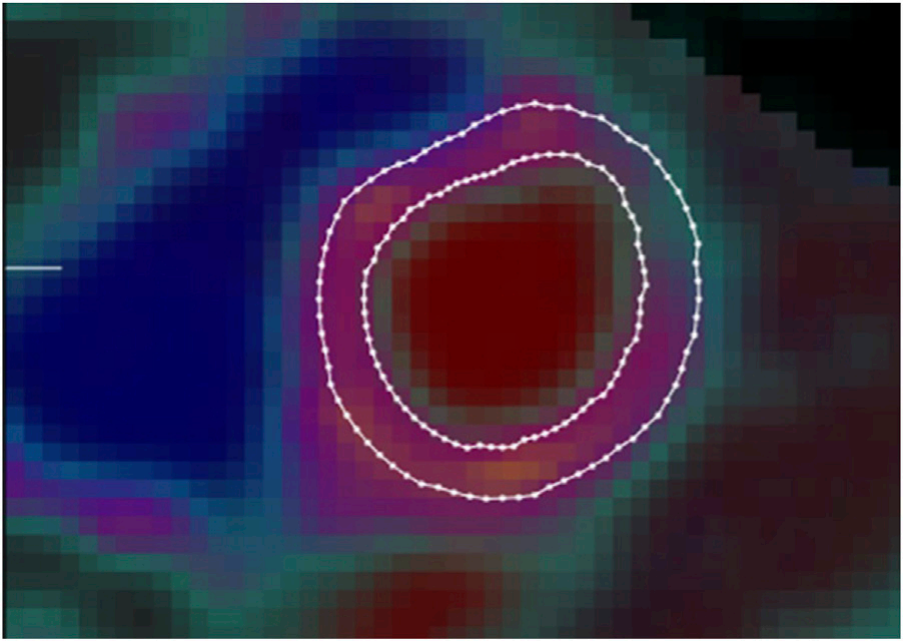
Example of left ventricular myocardial mass image as determined by ^15^O-H_2_O-PET/CT using semi-automatic segmentation of the left ventricular wall on parametric images of perfusable tissue fraction. Borders of endocardium (inner circle) and pericardium (outer circle) defined by delineated lines.
